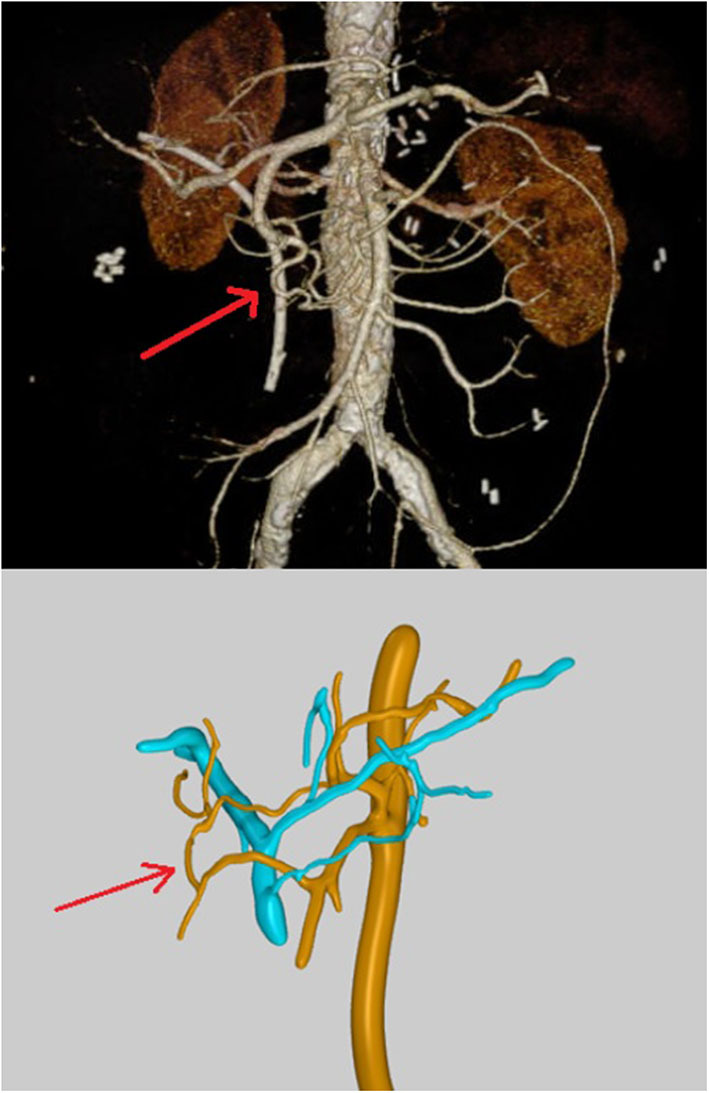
Upper panel: CT-angiography showing only a prominent Riolan anastomosis and possibly partly re-stenotic patch plastics of the celiac trunk and superior mesenteric artery; the red arrow points to a vessel that appears like a terminal branch of the gastroduodenal artery without any connection to the superior mesenteric artery; lower panel: virtual 3D-vascular and interactive reconstructions showing the Bühler anastomosis as connection between the superior mesenteric artery and the celiac trunk.
